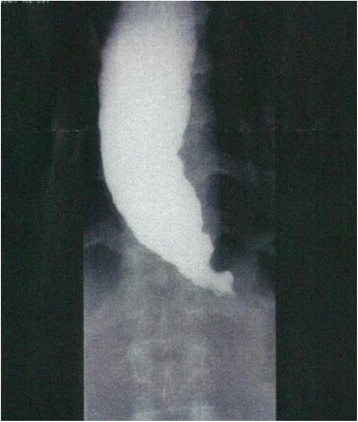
The esophageal radiography of case 2. The esophageal radiography shows dilation of the esophagus and a “bird-beak” appearance, which are characteristics of esophageal achalasia
